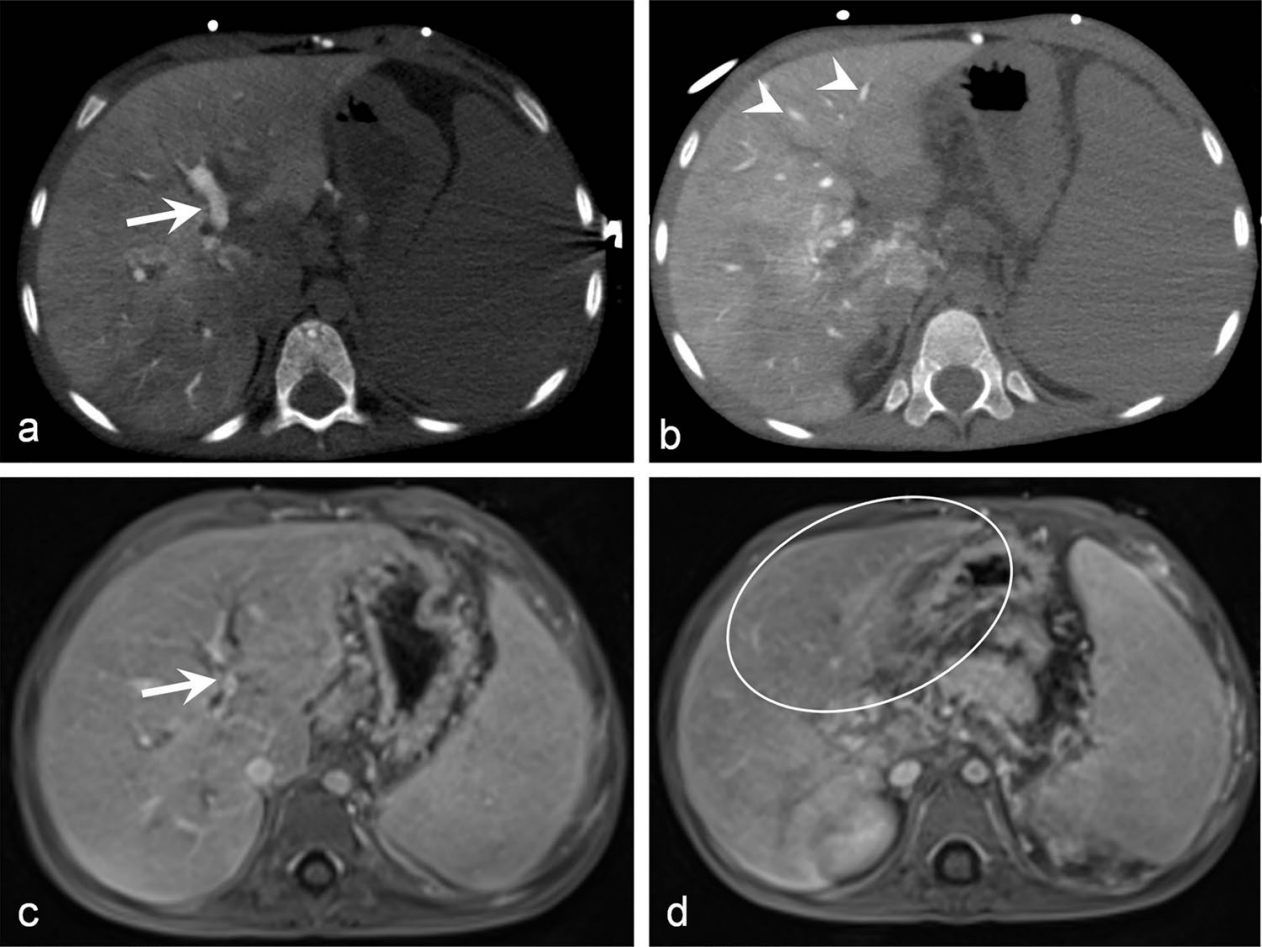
A 6-year-old male patient with EHPVO. Axial images of CT-AP (a, b) and CE-MR (c, d). The left central (arrows) and peripheral PV (arrowheads) were not confidently assessed in CE-MR, especially for the peripheral left PV CE-MR was non-diagnostic (circle in d).
